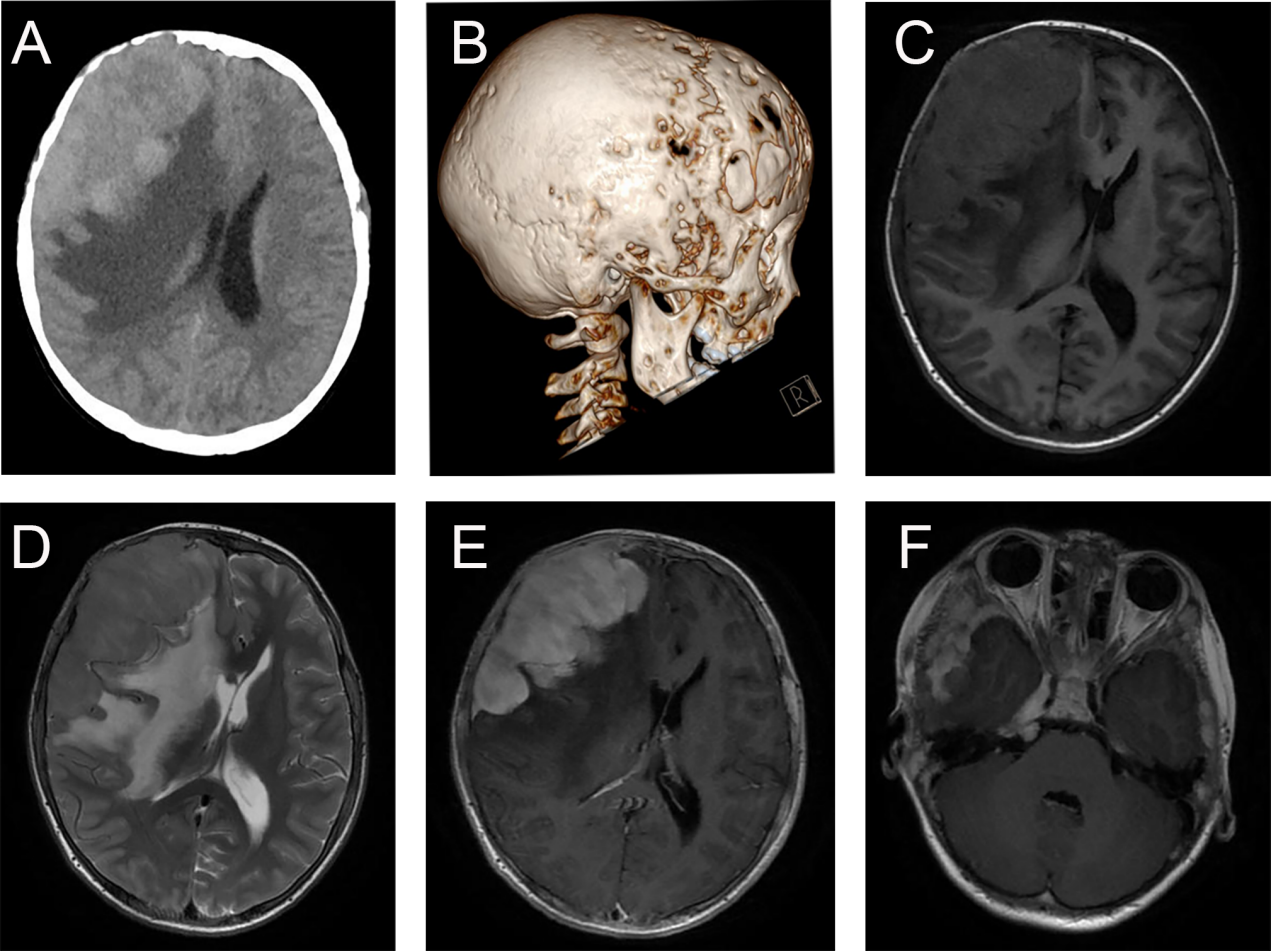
Typical imaging features of CNS-RDD (case 9). (A, B) CT images of axial cut and three-dimensional reconstruction showed destruction of the adjacent right frontal bone and a homogenous hyperattenuating mass with peripheral edema. MRI images with axial cuts show a homogeneous hypo- to the isointense mass of the right frontotemporal lobe on T1WI (C) and T2WI (D), with significant homogeneous enhancement and dural attachment (E). (F) Axial postcontrast T1WI showed CNS-RDD could also involve the right parasellar region.
